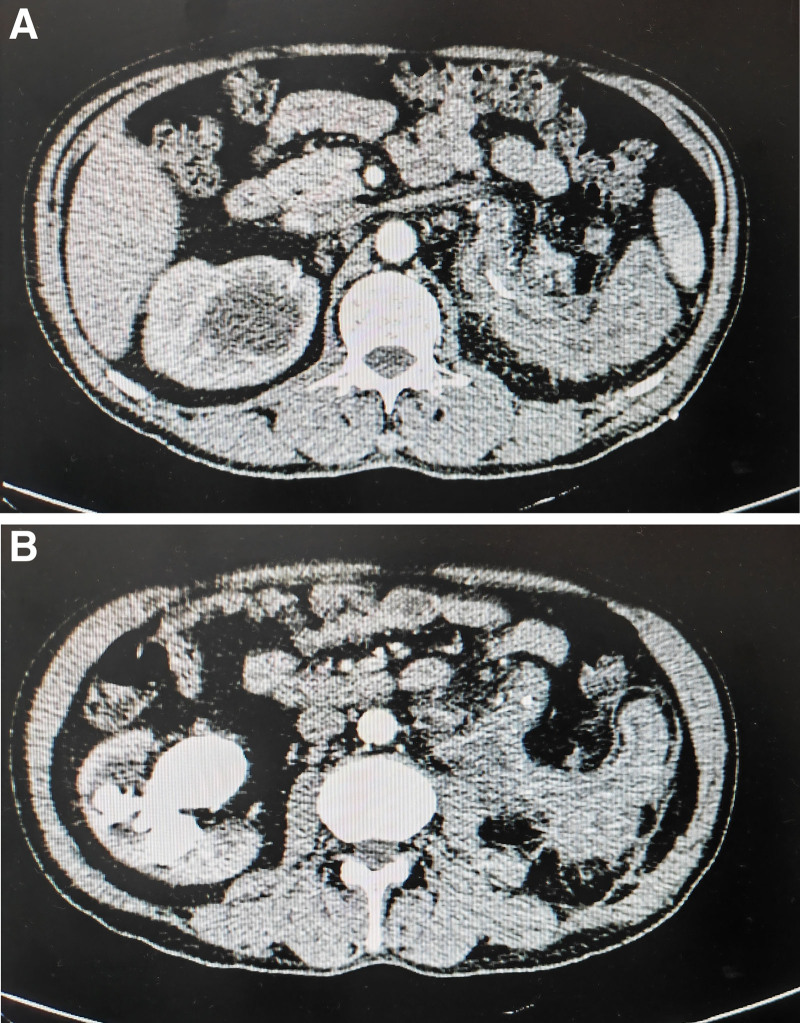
Computed tomography examination. (A) Patchy soft tissue density shadow in the left kidney region with heterogeneous enhancement, and patchy low density shadow without enhancement inside; (B) multiple lymph node enlargement around the lesion and beside the abdominal aorta, with a larger short diameter of about 1.1 cm, and heterogeneous thickening of the upper-middle segment of left ureter with obvious enhancement.
